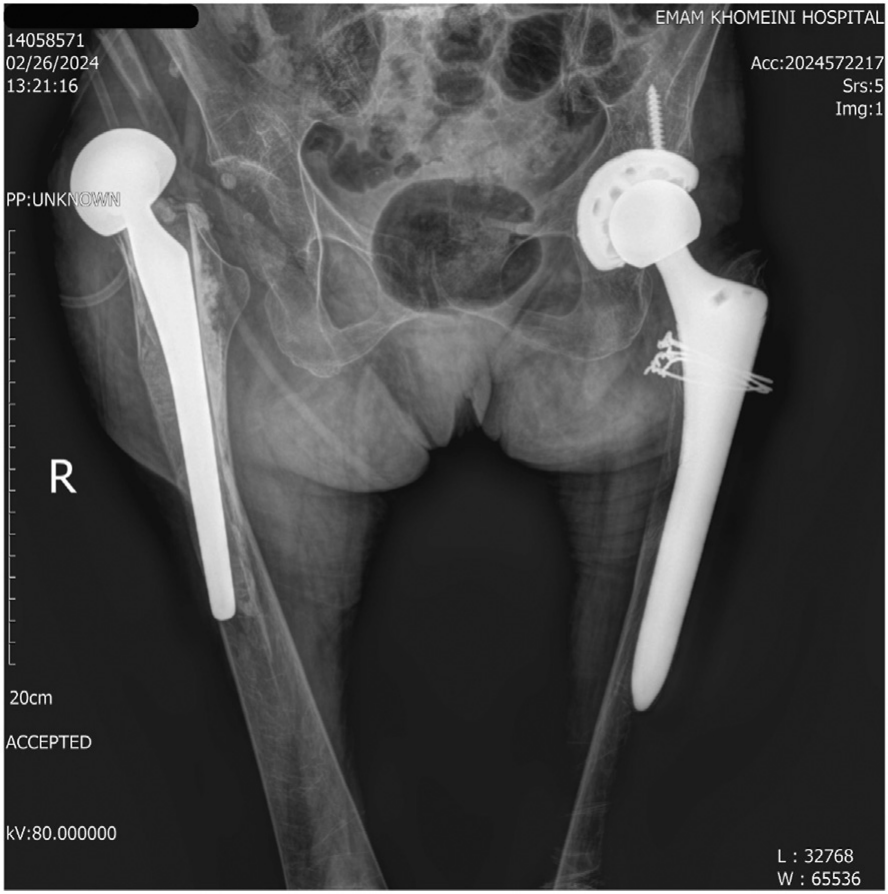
Preoperative radiograph of case 1.
